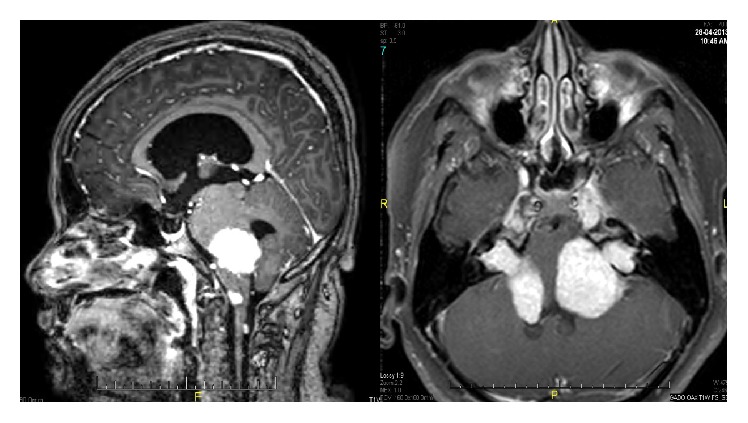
Brain MRI showing CP angle tumour, vestibular and trigeminal schwannoma, and bilateral concavity of cavernous sinuses.
